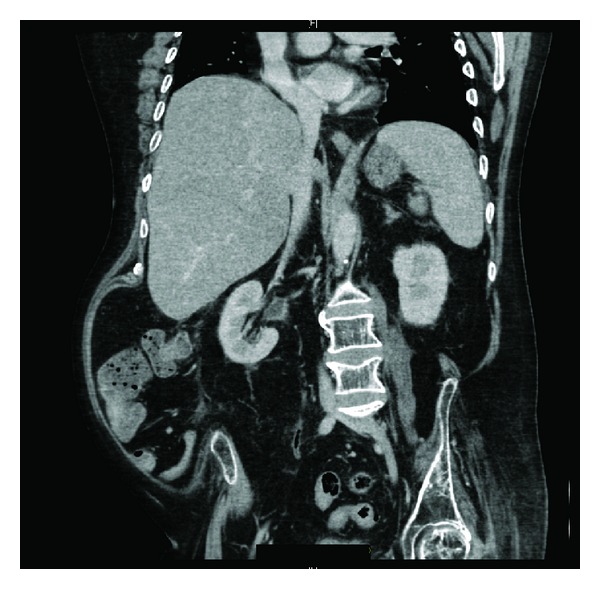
Coronal section of computed tomography of abdomen and pelvis revealed inflamed uroepithelium of right renal pelvis and gas in the lumen of right lower ureter.
